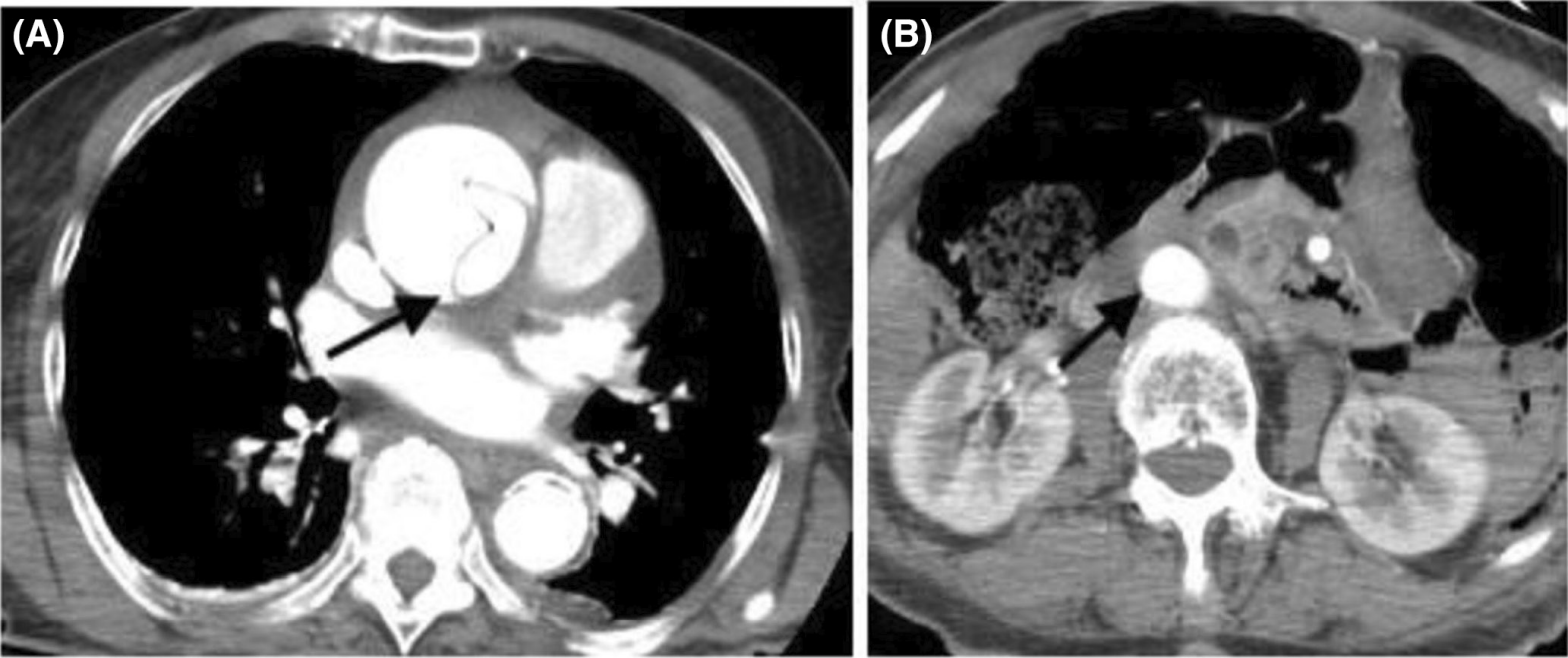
(A, B) CT angio of abdomen and pelvis with contrast showing type A aortic dissection.
